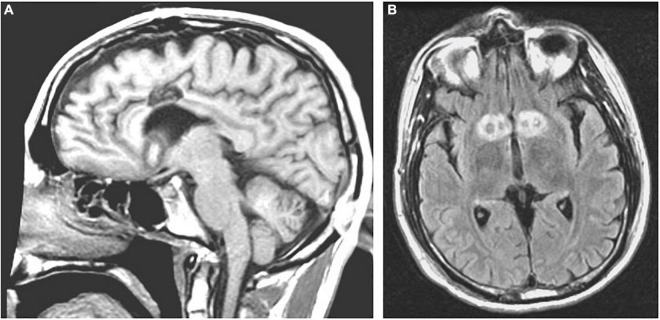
Limbic leucotomy. (A) Sagittal T1-weighted and (B) Axial FLAIR MRI of limbic leucotomy lesions.
